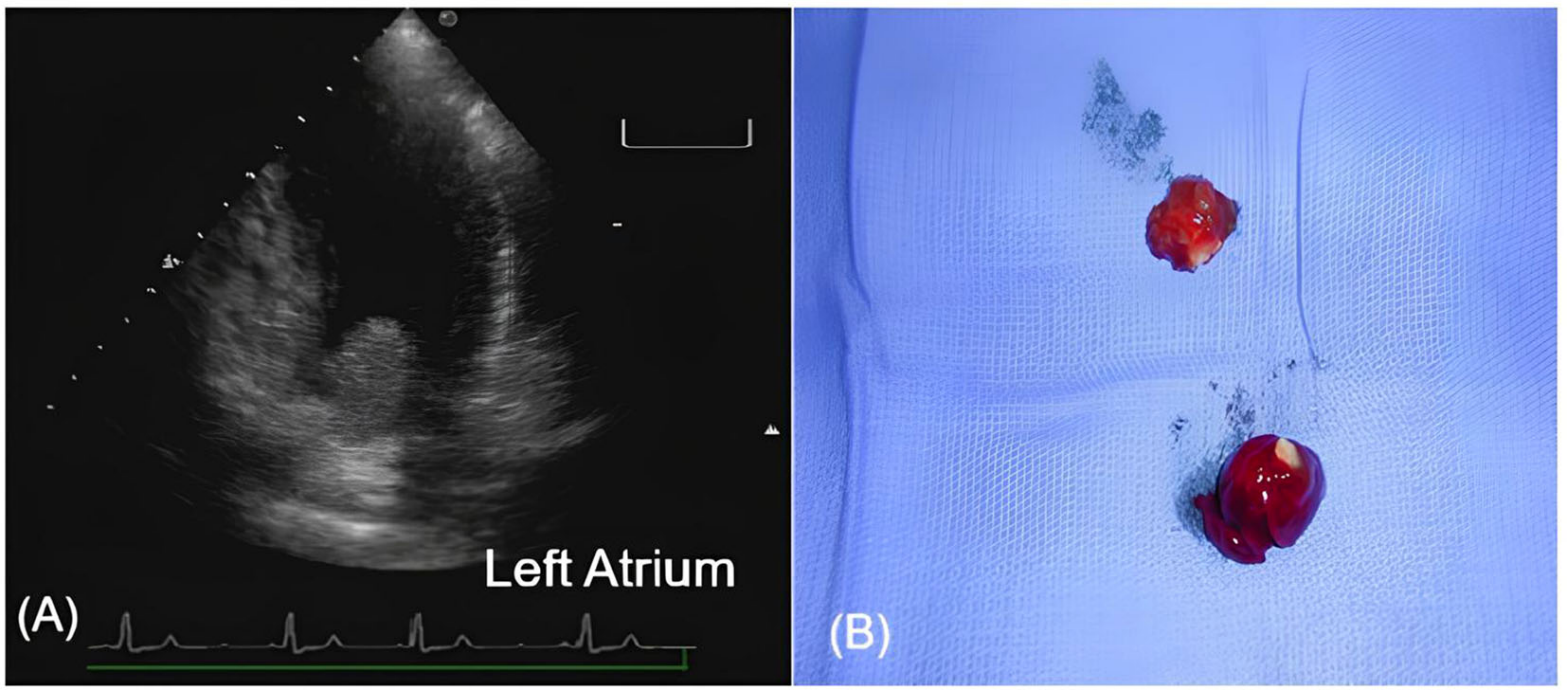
Recurrent left atrial myxoma originating from the interatrial septum. (A) Transthoracic echocardiography (apical four-chamber view) showing a mobile mass in the left atrium attached to the interatrial septum, measuring approximately 3.3 × 2.5 x 1.5 cm. (B) Gross pathology of the excised myxoma, showing a lobulated gelatinous tumor consistent with typical myxomatous appearance.
